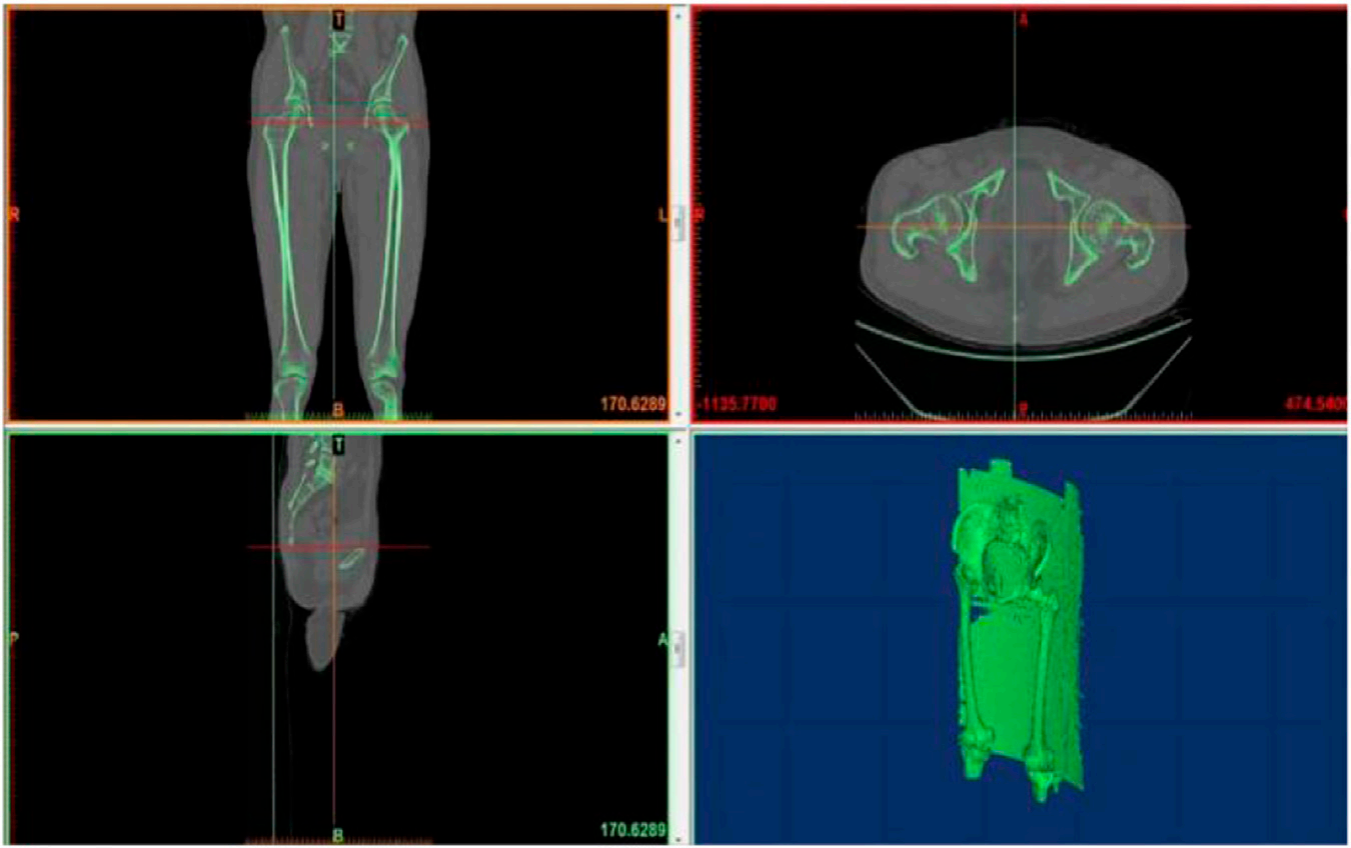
Femoral CT scan and 3D model image.
